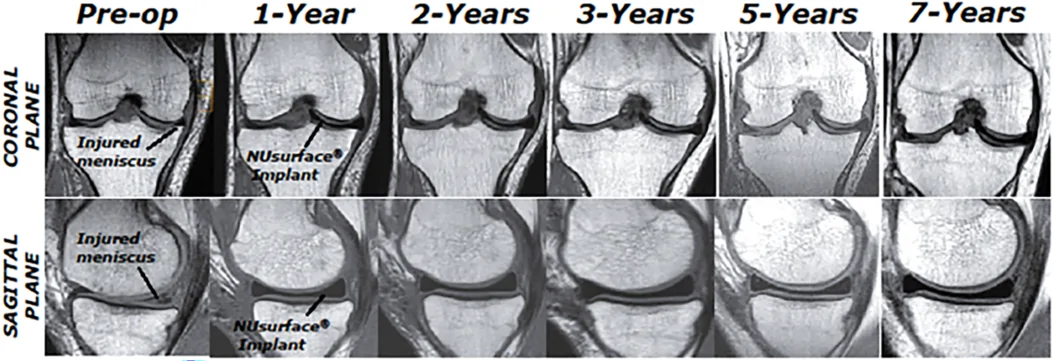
Representative coronal and sagittal MRI of the first NUsurface® patient, a 68‐year‐old male with unstable medial meniscus root tear.
